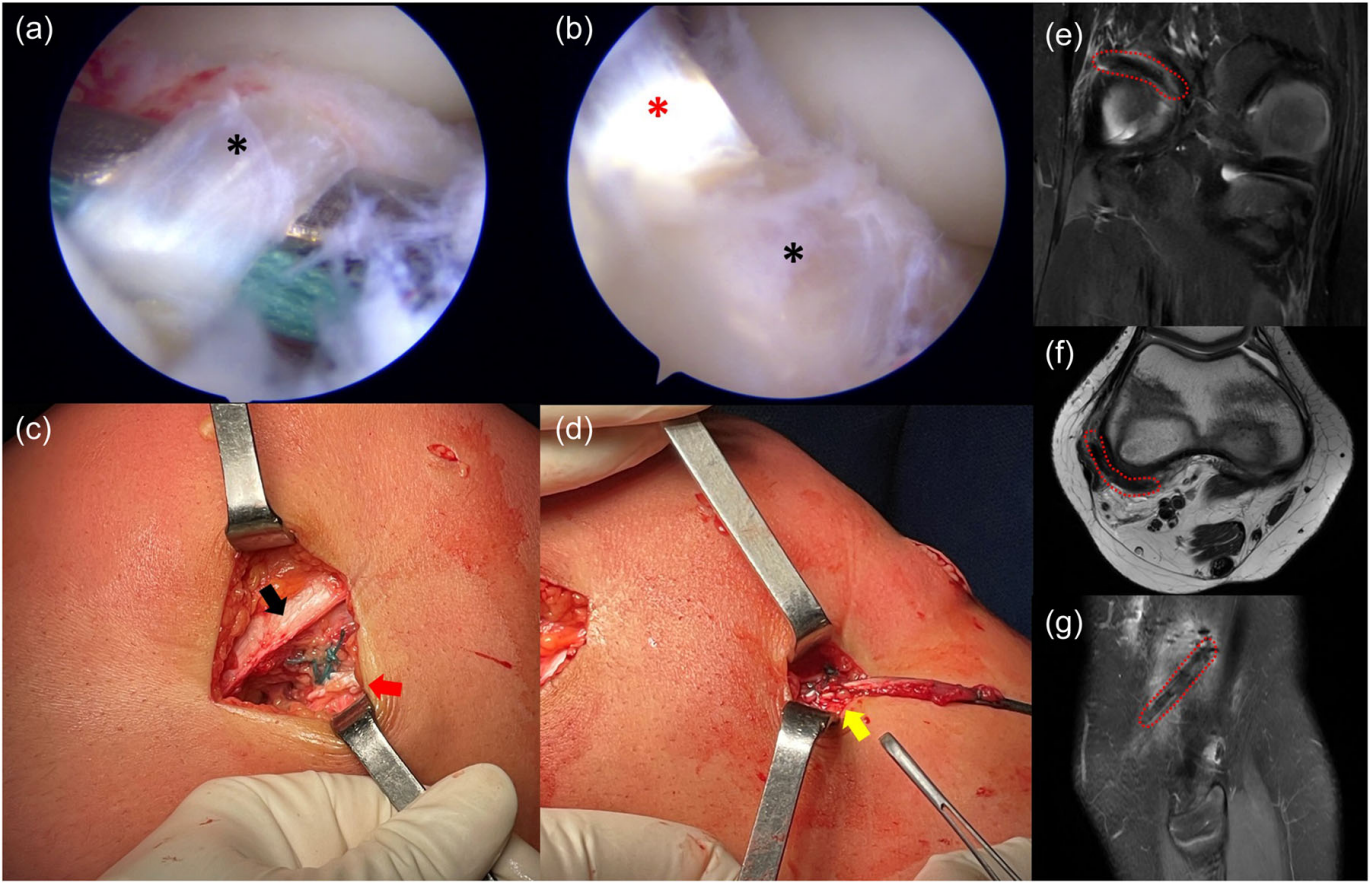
The Kelly clamp and a suture loop are passed below the intermeniscal ligament (black asterisk) (a). The hamstring graft (red asterisk) is passed below the intermeniscal ligament (black asterisk) as well (b). The graft (red arrow) is sutured extra‐articularly to the periosteum of the lateral distal femur with nonabsorbable sutures (black arrow) (c). The lateral tenodesis is sutured at the level of Gerdy's tubercle with nonabsorbable sutures (yellow arrow) (d). Post‐operative MRI shows the extra‐articular course of the graft above the lateral femoral condyle in the coronal (e) and axial (f) views, while the course of the lateral tenodesis can be seen in the sagittal view (g). MRI, magnetic resonance imaging.
